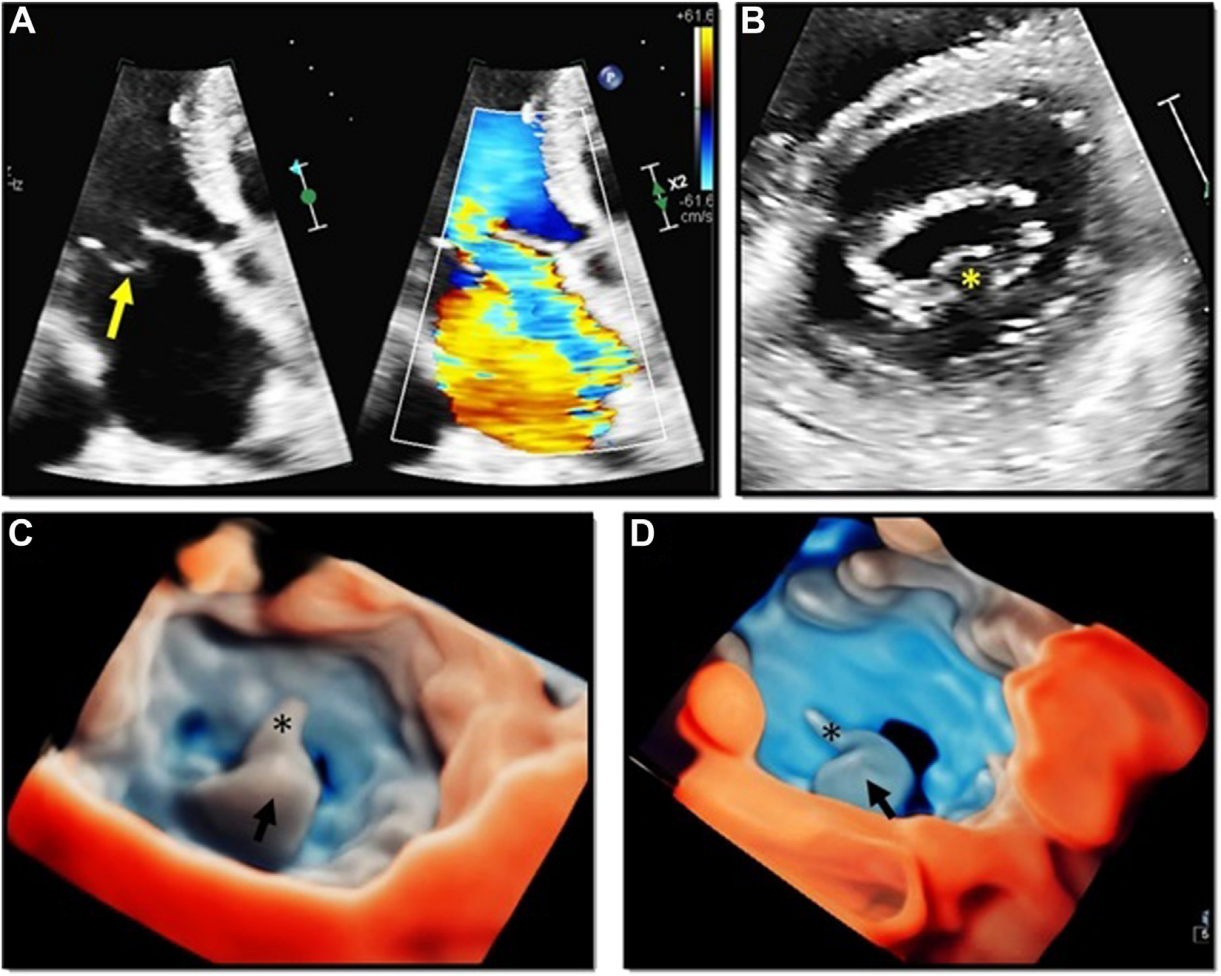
Enhanced definition of a MV P2 flail segment with the use of 3D-TTE imaging and TI rendering. Panel A shows a 2D-TTE apical 4-chamber view where a flail posterior leaflet *(left image, yellow arrow)* with a severe MR jet directed to the LA anterior wall *(right image)* can be appreciated. Panel B corresponds to a PSAX view, where the presence of a P2 flail segment was noticed *(yellow asterisk)*. Panels C and D show the advantages of 3D-TTE TI rendering, which provides optimal visualization of the flail P2 segment *(black arrow)* and the ruptured chordae *(black asterisk)* on an en face view of the MV.
